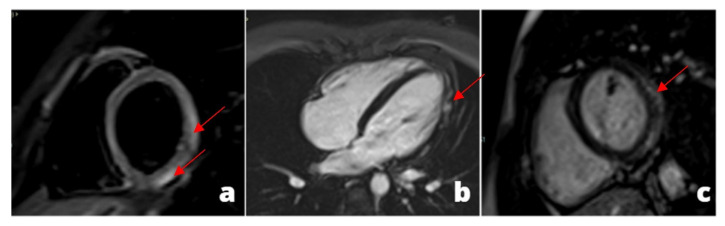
A young adult presenting at emergency department with acute chest pain, subtle ST alterations in the lateral wall of the left ventricle and cardiac enzymes elevation. Cardiac MRI was performed 48 h later demonstrating edema in the inferior-lateral wall of the left ventricle (a) associated with hyperemia in early gadolinium enhancement (b) and post-inflammatory fibrosis using late gadolinium enhancement sequences (c).
